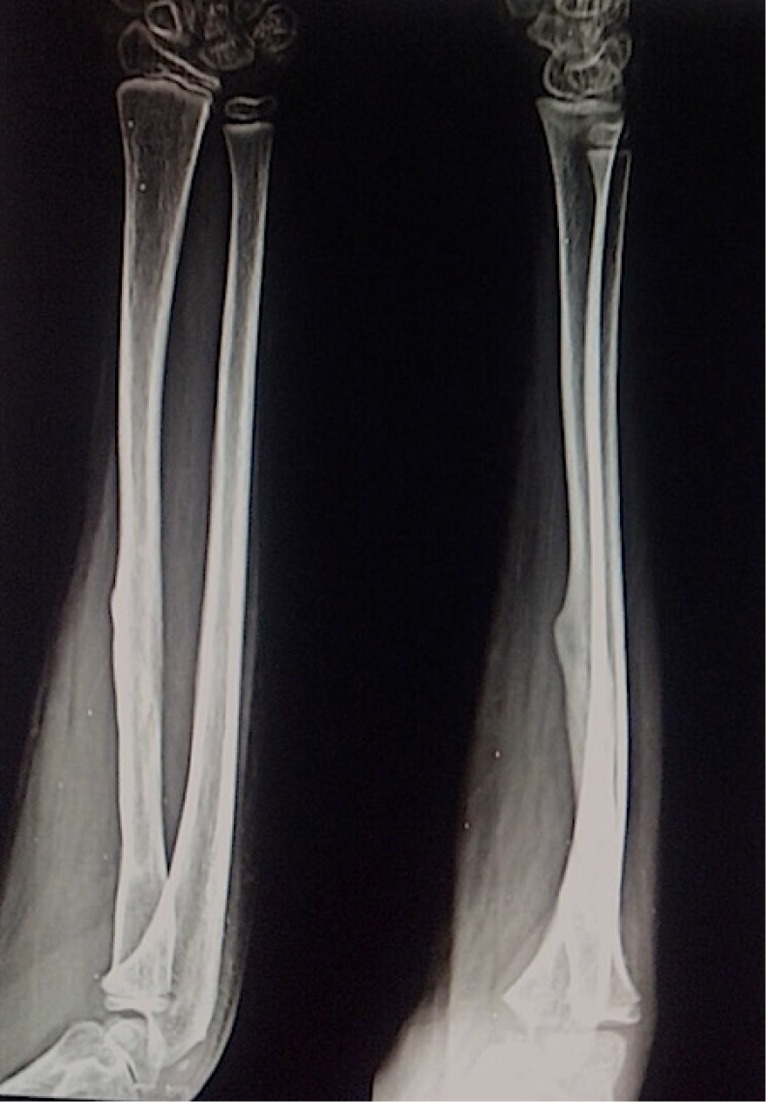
Two years follow-up roentgenograph showing fully incorporated graft into the host bone.
